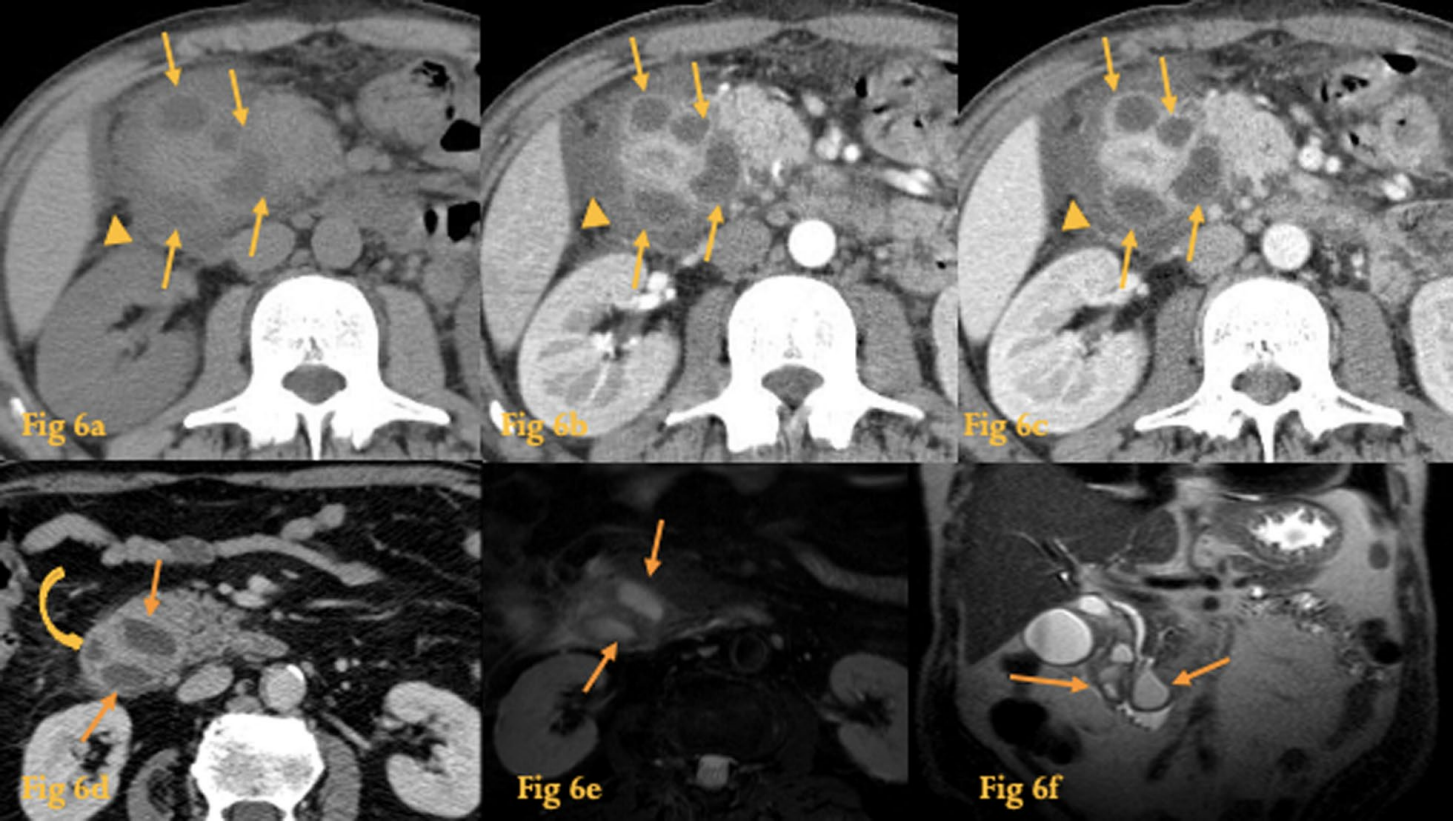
Cystic dystrophy of the duodenal wall. Abdominal CT of a patient with abdominal pain (a–c)—there is parietal thickening and several cystic lesions surrounding the duodenum (arrows), best evaluated after contrast administration (b, c). These cysts have a preferential localization in the mesenteric side. Densification of the periduodenal fat and a small amount of fluid surrounding the duodenum (arrowheads) is also seen. Abdominal CT of another patient (d)—duodenal intramural cyst-like lesions with fluid density (arrows). The cysts narrow and shift the duodenal lumen to the right. In abdominal MR (f, e), cystic lesions (arrows) present high signal intensity on T2w images
